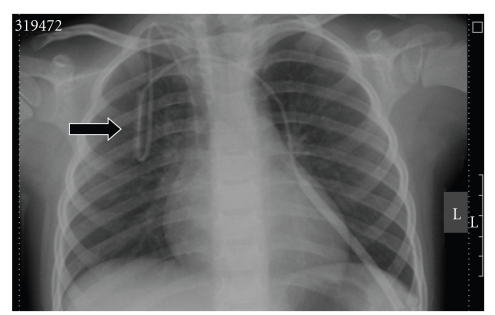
X-ray with correct position of the CVC, repaired area indicated by arrow.
